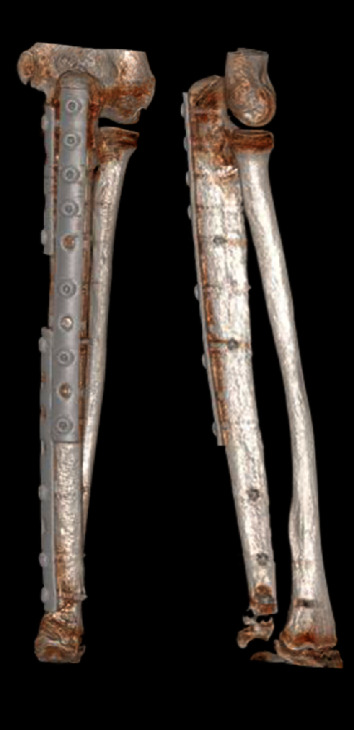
The same patient mentioned in Figure 1. CT scan performed 20 months after the surgery showing good healing at the proximal host-allograft junction and preservation of the distal ulnar physis.
